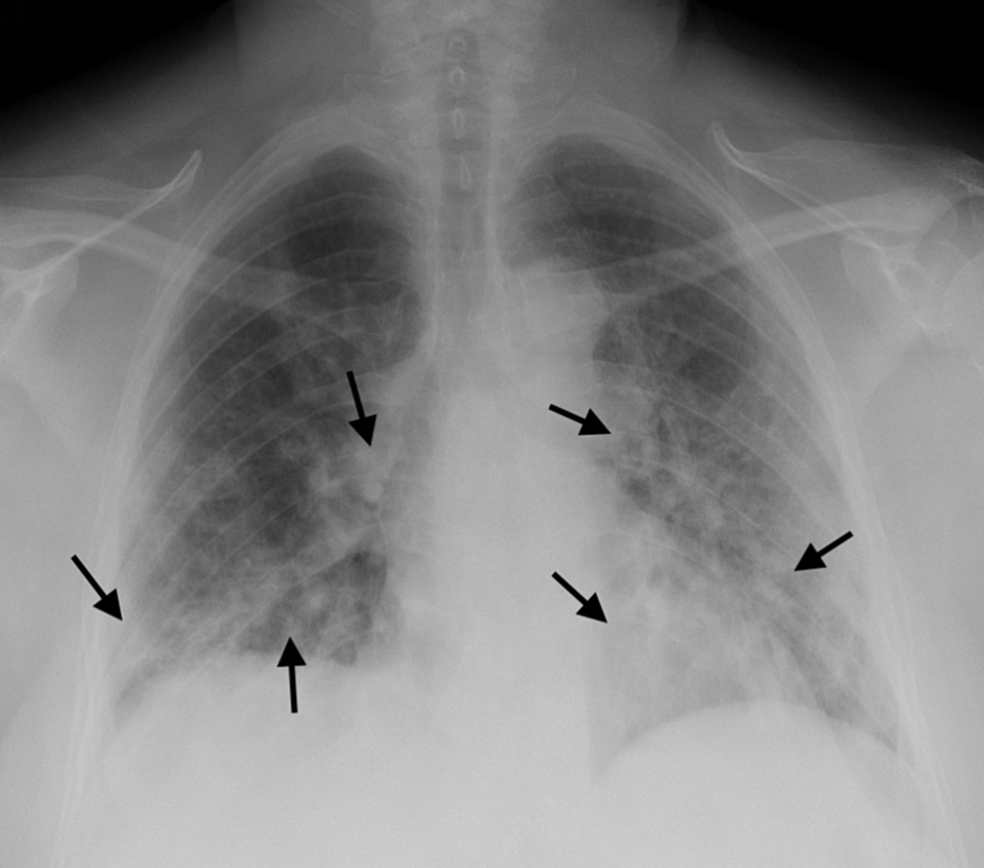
Chest radiograph showing bibasilar predominant multifocal opacities (arrows).
